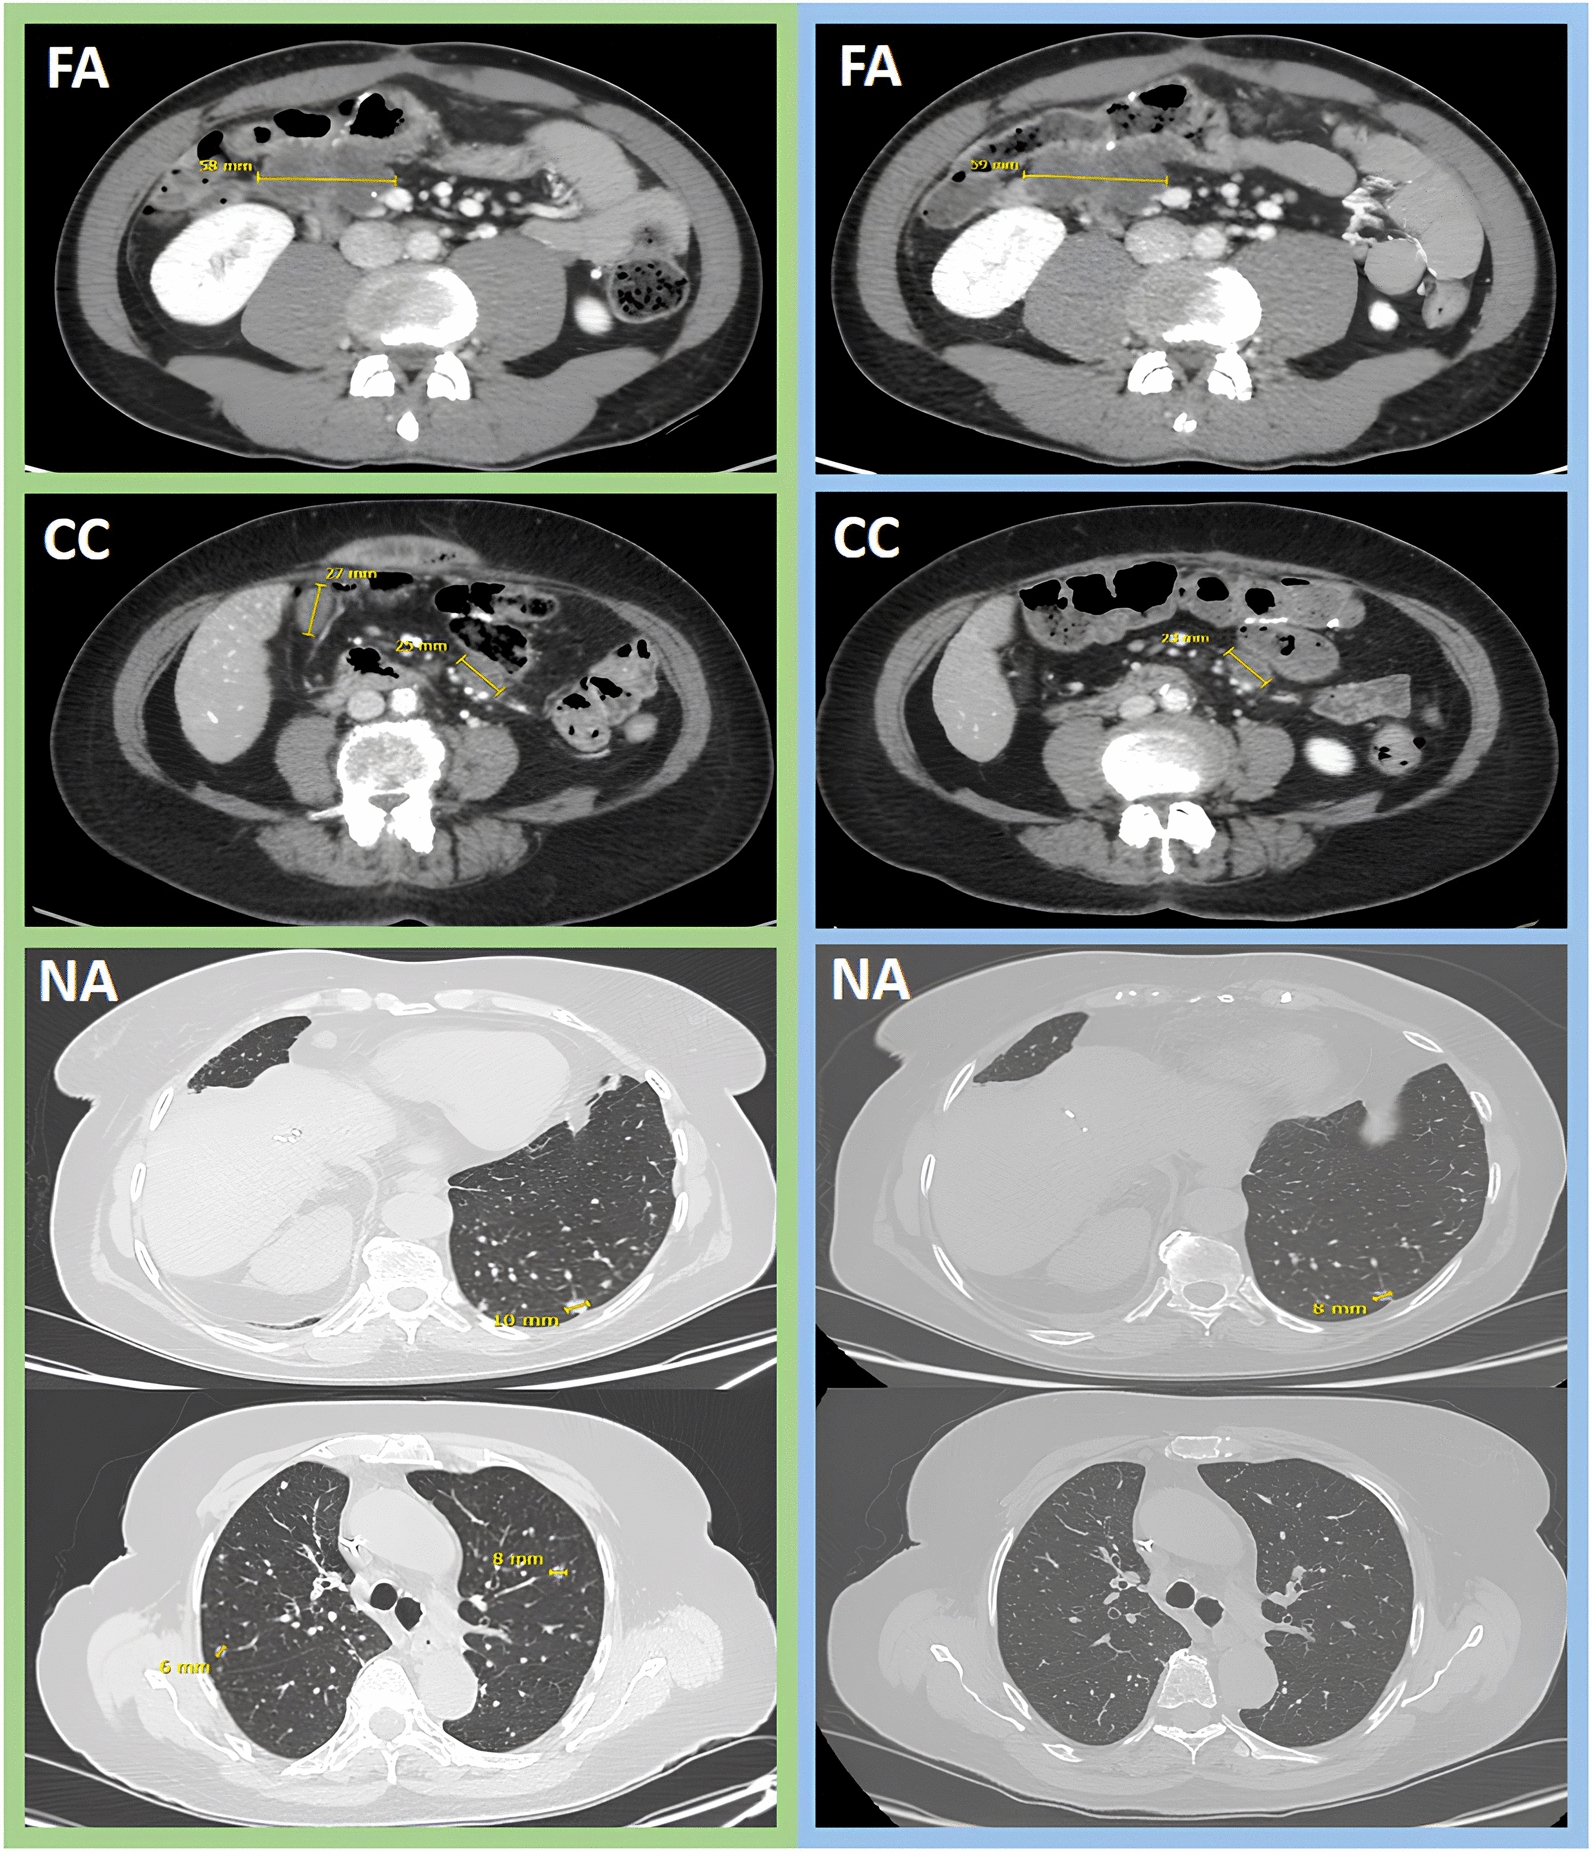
Representative computed tomography (CT) restaging images depict FA, CC, and NA patients with long-lasting disease control (FA, CC, and NA represent patients' initials). Baseline CT scans at TAS-102 treatment initiation are outlined in green frames, while CT scans at the last available restaging (third for FA and CC patients, second for NA patients) are framed in blue. Measurable lesions in the peritoneum (FA: 58 mm vs 59 mm), abdominal lymph nodes (CC: 22 mm vs 0 mm; 25 mm vs 23 mm), and lungs (NA: 10 mm vs 8 mm, 6 mm and 8 mm vs 0 mm and 0 mm) are delineated by their longest diameters, with measurements in millimeters reported within the images
